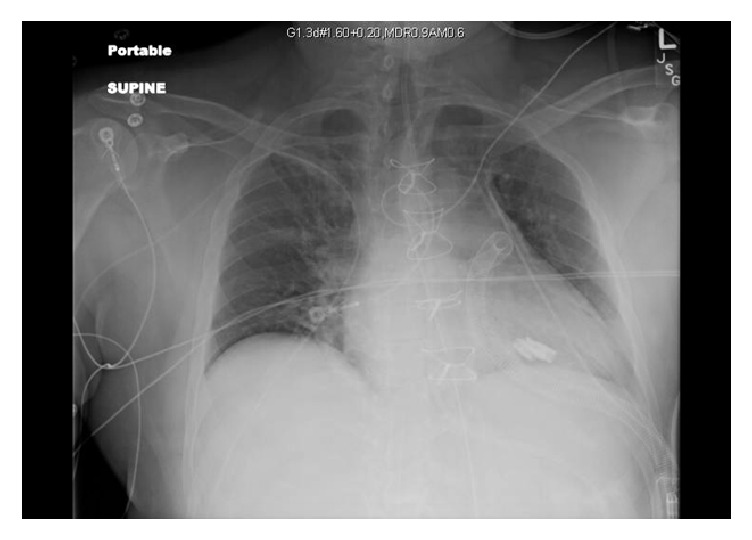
Portable chest X-ray, supine.
